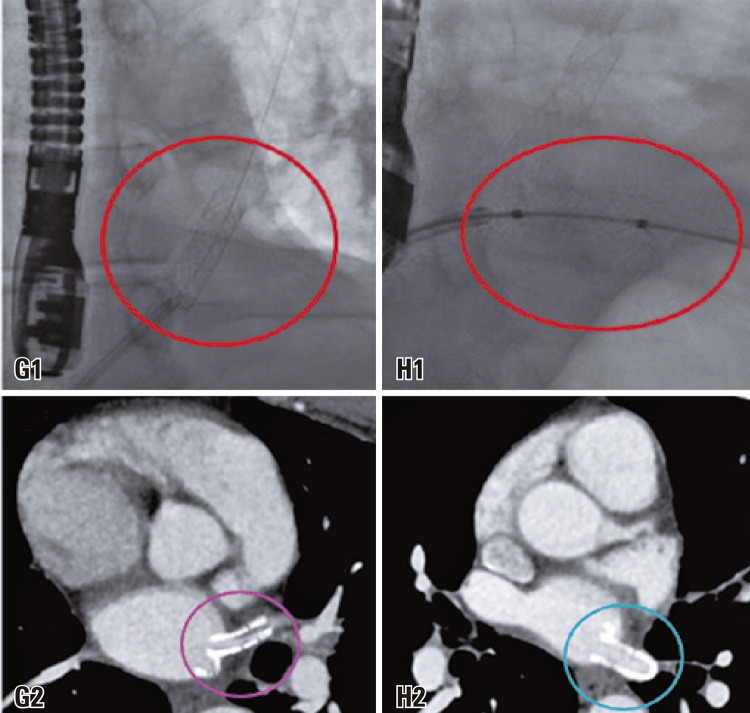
Stent placement within the upper pulmonary vein ostia (G1), and lower pulmonary vein ostia (H1). After 6 months, a subsequent computed tomography pulmonary angiogram examination reveals restenosis in the left upper pulmonary vein (pink circle in image G2) while demonstrating a patent inferior pulmonary vein with preserved caliber (blue circle in image H2)
